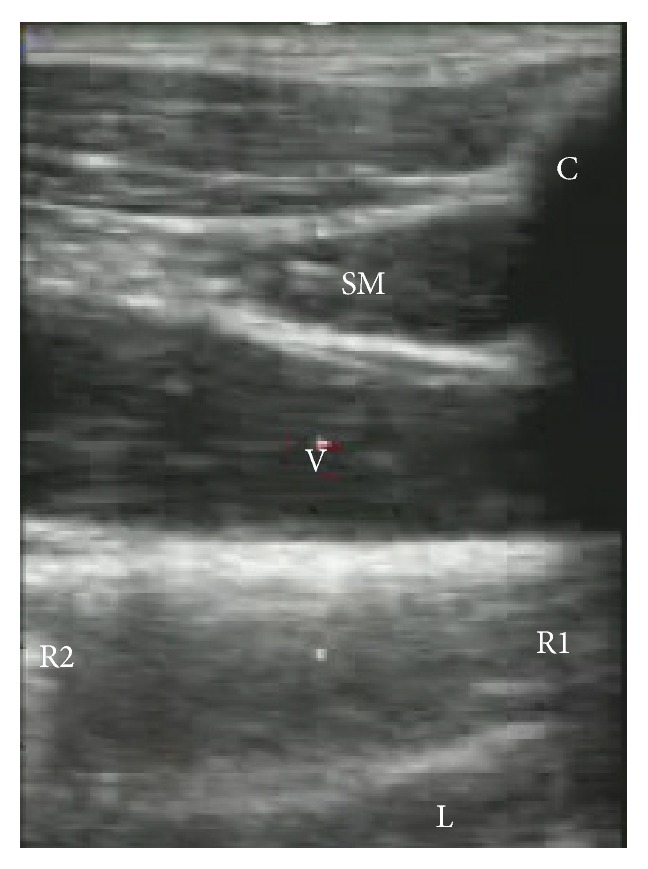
Ultrasound view of the subclavius muscle. SM: subclavius muscle, C: clavicle, V: subclavian vein and/or infraclavicular axillary vein, R1: first rib, R2: second rib, and L: lung.
